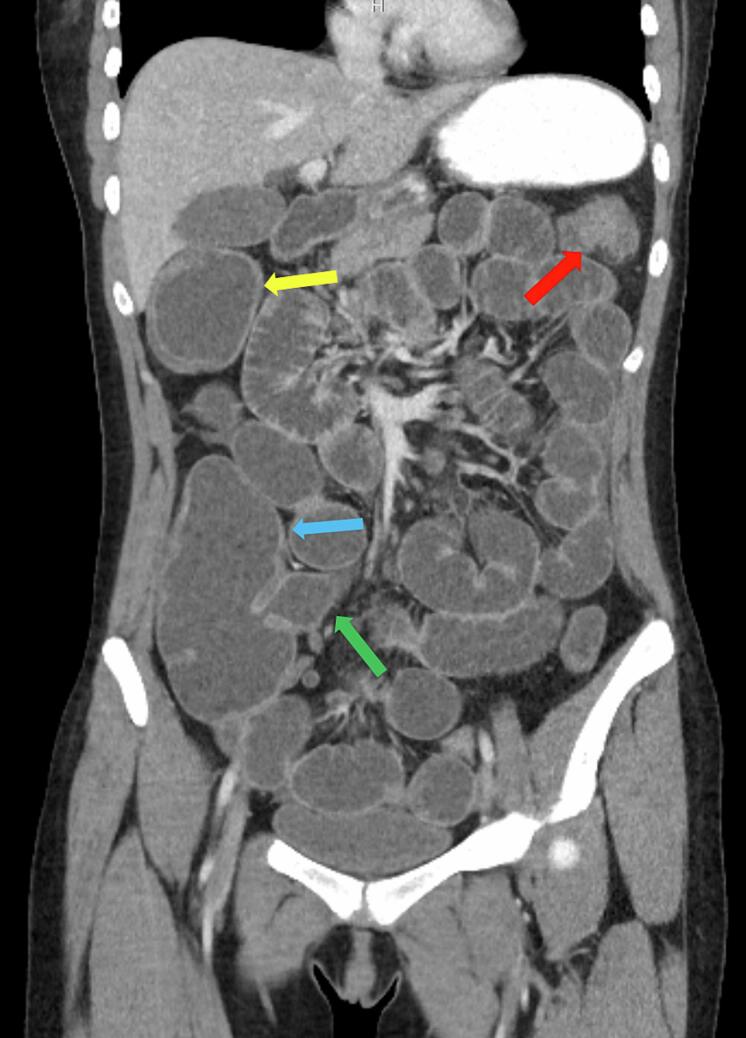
Coronal CT of the abdomen and pelvis showing distended hepatic flexure with wall thickening (yellow arrow), distended ascending colon with wall thickening (blue arrow), focal narrowing at the splenic flexure (red arrow) with distended terminal ileum (green arrow).
